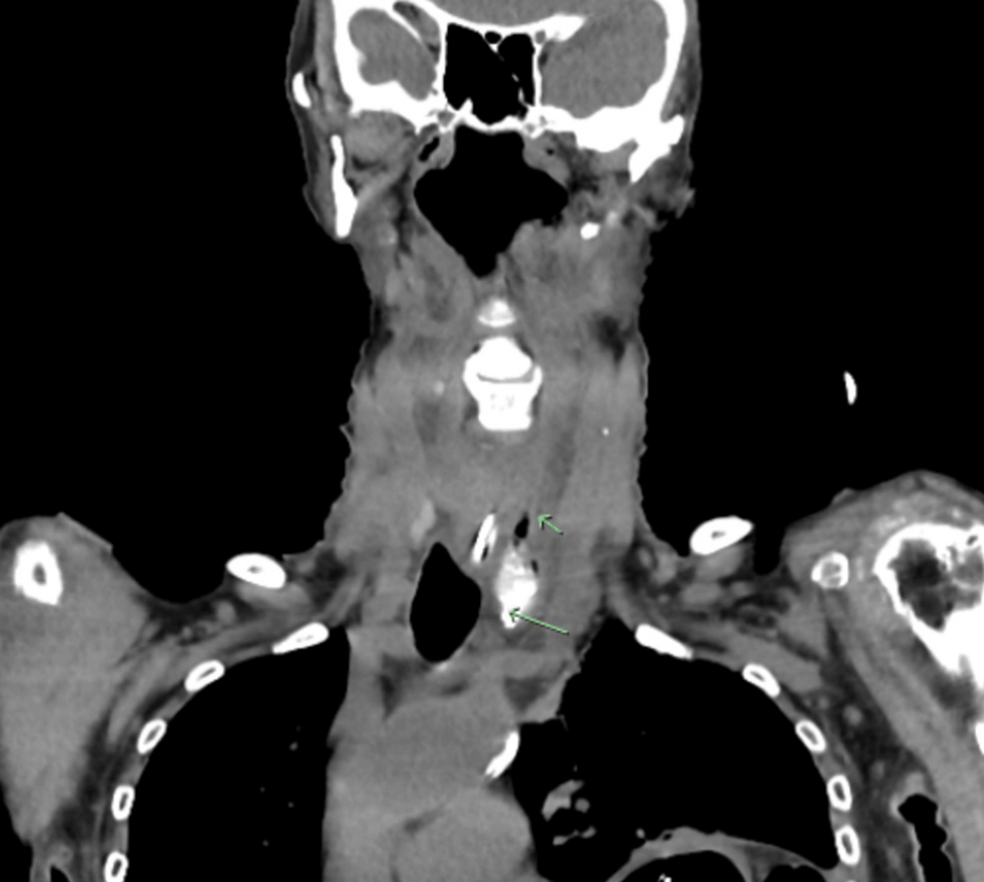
Coronal view of a non-contrast computed tomography of the neck which confirms a left parapharyngeal collection with free gas (green arrows) and pooling of the previously swallowed modified barium contrast material.
